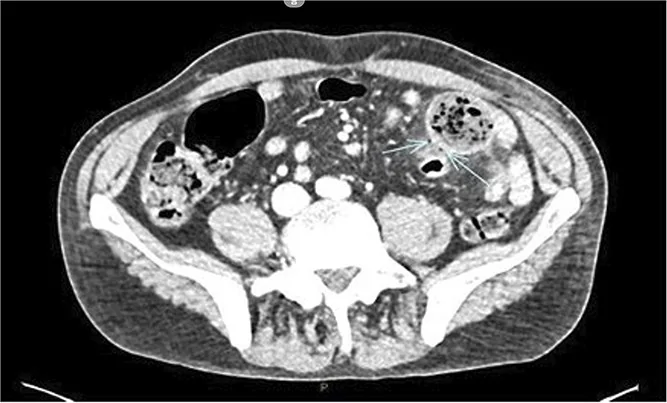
Contrast-enhanced CT abdomen demonstrating dilated small bowel loops proximal to a transition point in the distal ileum.
